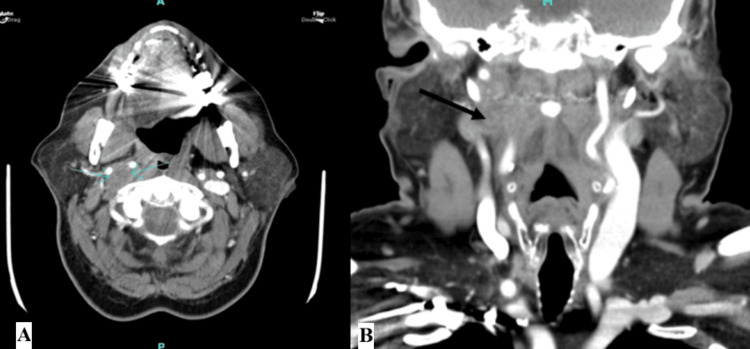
(A) Axial CT showing a defined hypoechoic mass after treatment with SBRT. (B) Coronal CT showing the same lesion without further progression from initial imaging pretreatment
